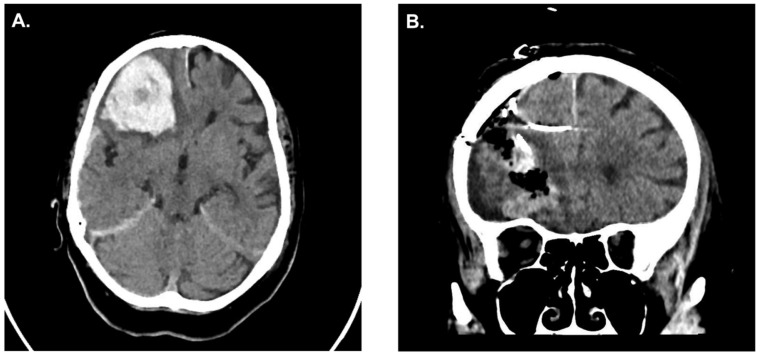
(A) Preoperative CT scan of a patient with right frontal intracerebral hemorrhage. (B) Postoperative CT scan showing hematoma evacuation with simultaneous ICP monitoring. ICP, intracranial pressure.
